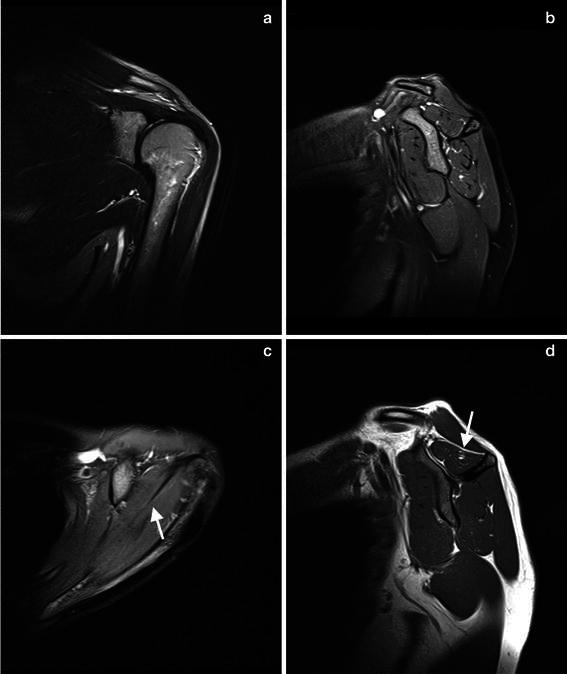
Magnetic resonance imaging performed 29 months after trauma. a T1 short-tau inversion-recovery coronal image shows complete disappearance of edema, even though the muscle is hypotrophic. b Proton-density fat-saturated sagittal and c axial view confirms the reduction of muscle volume and shows a small intramuscular scar in the posterior side of the musculotendinous junction. The intramuscular septum is solid and stretched (*arrow*). d Grade 1 fatty degeneration clearly visible on T1 sagittal view (*arrow*)
